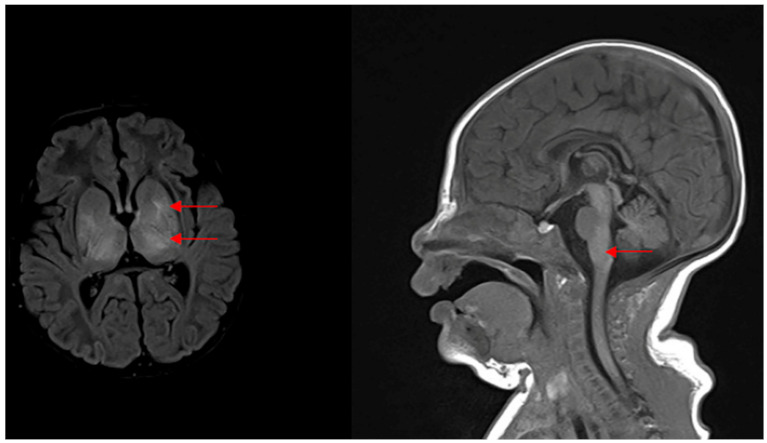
The brain magnetic resonance imaging (MRI) in P9 (Table 4) exhibited bilateral abnormalities of the basal ganglia, thalamus, and brainstem, which are involved in the nucleus of vestibulocochlear nerve with clinical stage III hypoxic–ischemic encephalopathy and profound hearing impairments.
